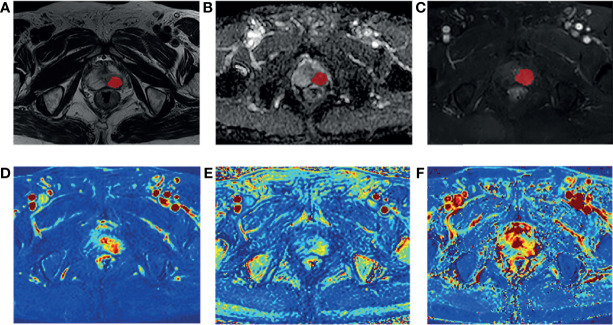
A 80-year-old man diagnosed with csPCa in TZ (PSA,59.90 ng/mL; biopsy GS, 3 + 4 = 7). Example segmentations (red masks) of the tumor overlaid on on axial T2-weighted imaging (T2WI) (A), apparent diffusion coefficient (ADC) map (B), and dynamic contrast-enhanced MRI (DCE-MRI) (C). The ROIs were selected based on the enhanced T1WI and then matched to Ktrans map (D), Kep map (E), and Ve map (F).
